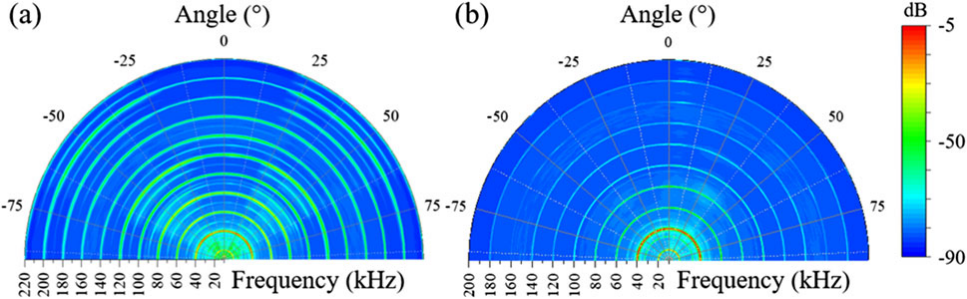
a Polar plot (shown in false color) of the dependence of the ultrasound emission spectra generated by a 10 $$\times $$ 10 array of microplasma jets. The abscissa gives the emission frequency (in kHz), and the color scale at right provides relative intensities. This hemispherical representation assumes the exit plane for the jet array coincides with the abscissa and the microjets are propagating upward; b data similar to those of a for the static array shown in operation in He in Fig. 1d
